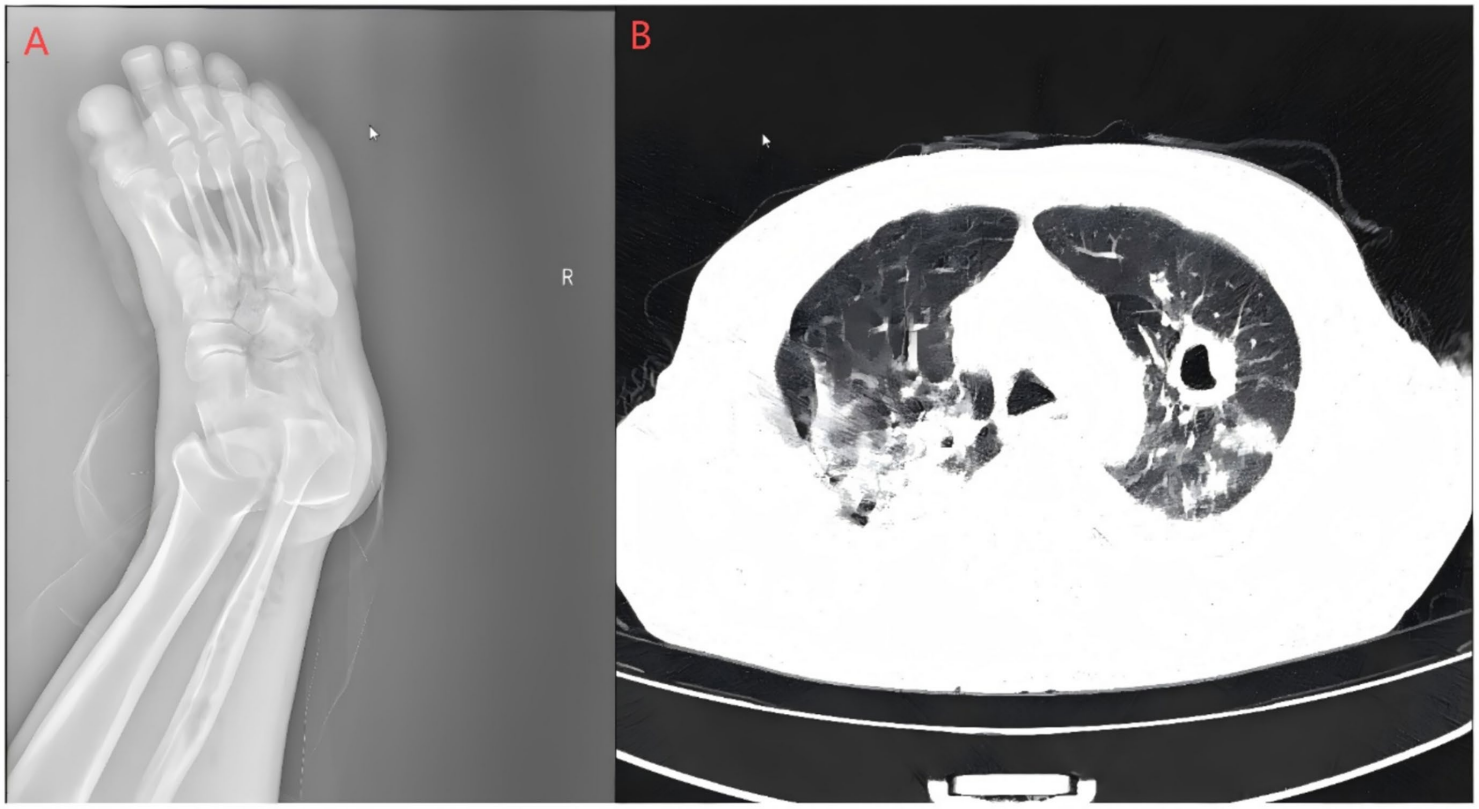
Right foot X-ray and pulmonary CT scan. (A) Bone resorption and destruction can be seen in the first toe of the right foot, and the soft tissue of the right foot is swollen and broken with gas accumulation; (B) polymorphic lung infections with cavity formation bilaterally and a small amount of pleural effusion on both sides. The Supplementary images are available upon reasonable request.
